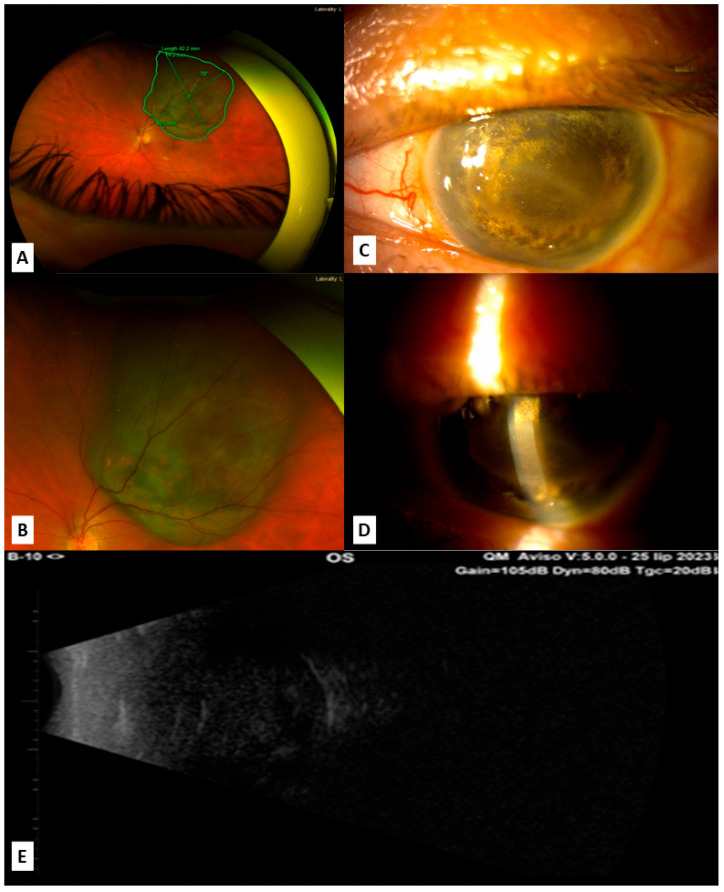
Images showing the left eye of the patient; (A) measurement of the melanoma at the moment of first admission; (B) melanoma at the moment of first admission; (C,D) slit lamp examination of the left eye (OS) in the moment of SO appearance in OD, 2 years after initial tumor diagnosis: complete shallowing of the anterior chamber due to tumor masses in the posterior segment of the eye; (E) ultrasound at the moment of SO appearance shows that left eye is completely filled with tumor masses.
